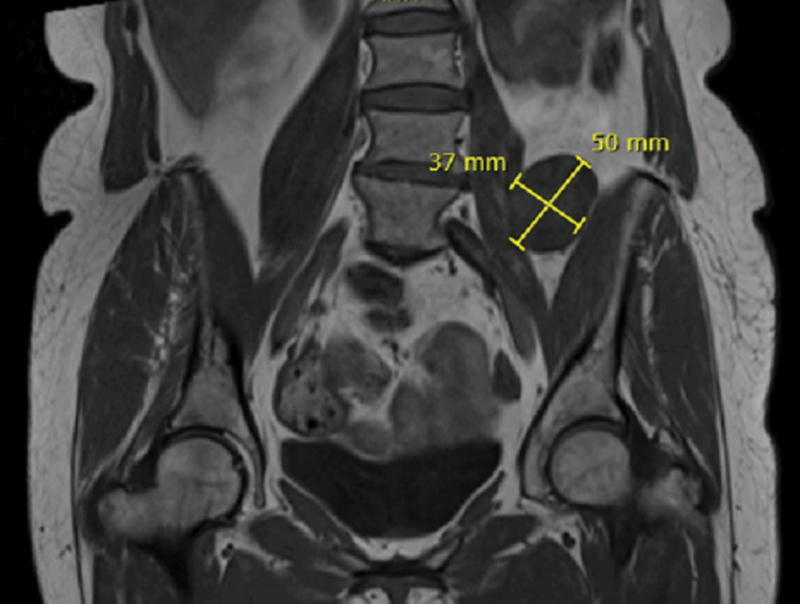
MRI (coronal plane) – T1- weighted sequence: hypointense ovoid, nodular paraspinal mass, closely related to the left L4 nerve root and located between the psoas and iliacus muscles.
